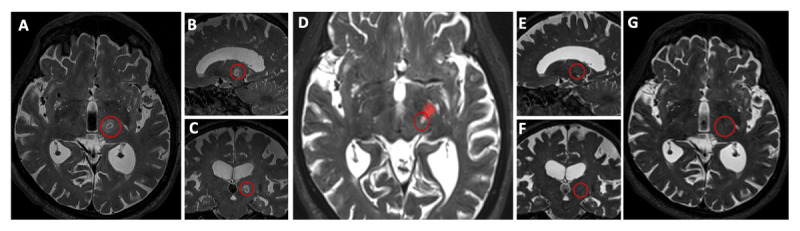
(A–C) Axial, sagittal, and coronal high-resolution T2-weighted MRI acquired 3 days after magnetic resonance-guided focused ultrasound thalamotomy demonstrate a hyperintense lesion with central hypointensity, consistent with necrosis and surrounding edema. (D) Diffusion tractography overlay reveals proximity of the lesion to the corticospinal tract. (E–G) Follow-up imaging at 3 months shows decreased lesion size on axial, sagittal, and coronal views, respectively.
